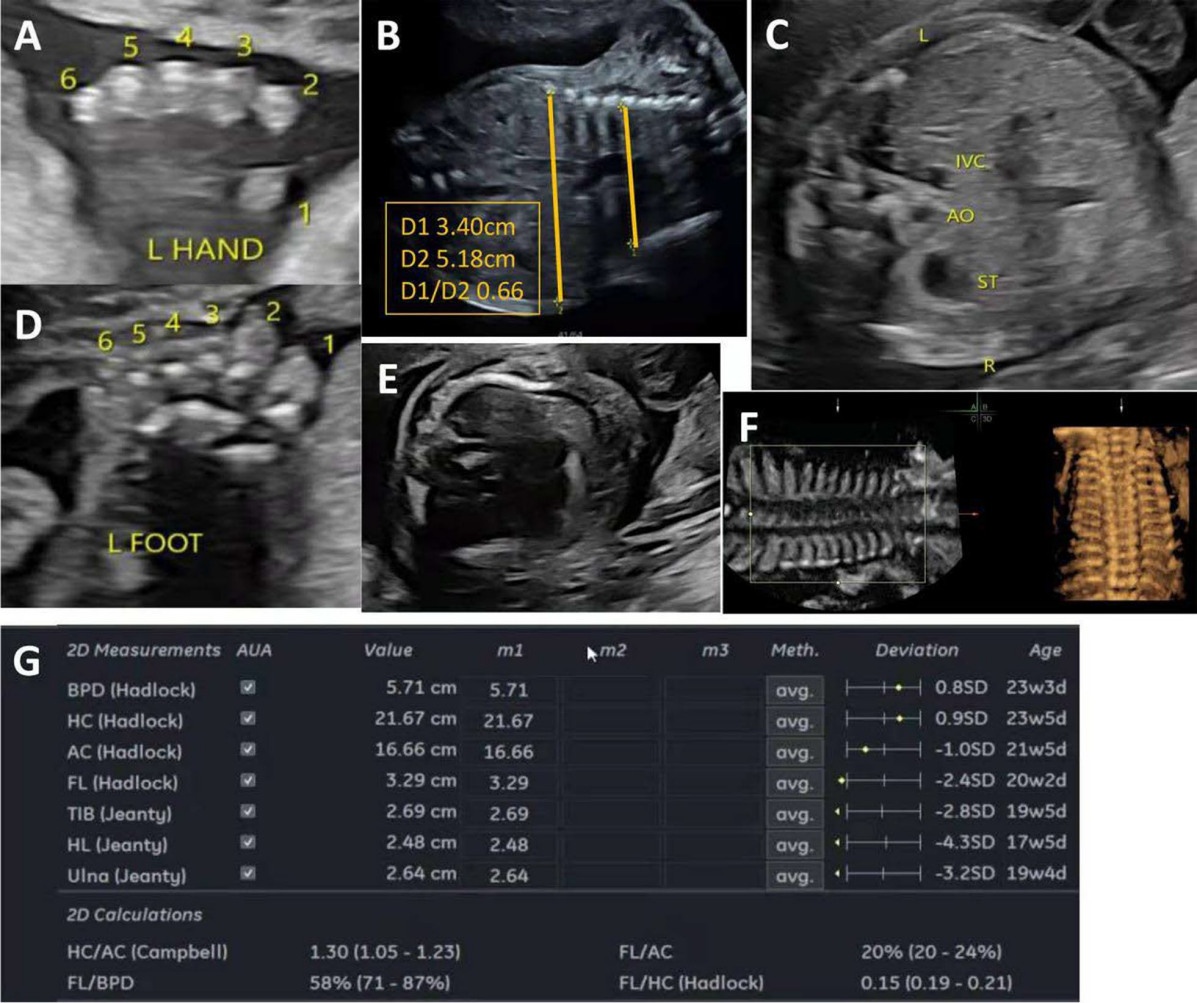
The sonographic results of the foetus. A, D Polydactyly, and syndactyly. B The thoracoabdominal transverse diameter ratio was 0.66. C The abdominal organs of the foetus were in the opposite position of normal, i.e., in a mirror image position, with the gastric vacuole and descending aorta on the right, the apex of the heart facing right, and the gallbladder, and inferior vena cava (IVC) located on the left side. E, F: Abnormal rib curvature and narrow thorax. G Ultrasound measurements (Hadlock and Jeanty) based on gestational age. The FL, TIB, HL and ulnar values were below 2.4 SD, 2.8 SD, 4.3 SD and 3.2 SD, respectively, compared with the average. Abbreviations: L, left; R, right; IVC, inferior vena cava; AO, aorta; ST, stomach; BPD, biparietal diameter; HC, head circumference; AC, abdominal circumference; FL, femur length; TIB, tibia length; HL, humerus length
